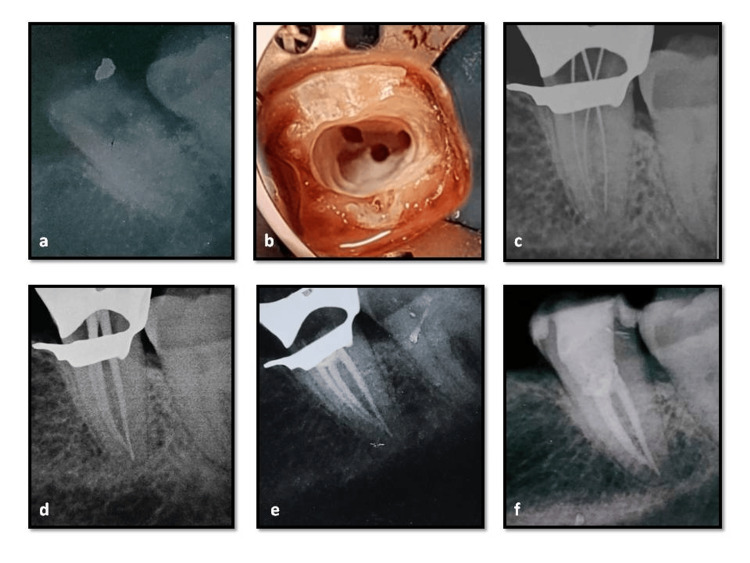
Case report-3 a) Preoperative radiograph; fused mesial and distal roots; b) access opening, three discrete canal orifices arranged in a C-shaped configuration; c) working length radiograph, narrow isthmus present between the mesial canals; d) master cone radiograph, all three canals merging at the apical third; e) obturation radiograph; f) postoperative radiograph.
